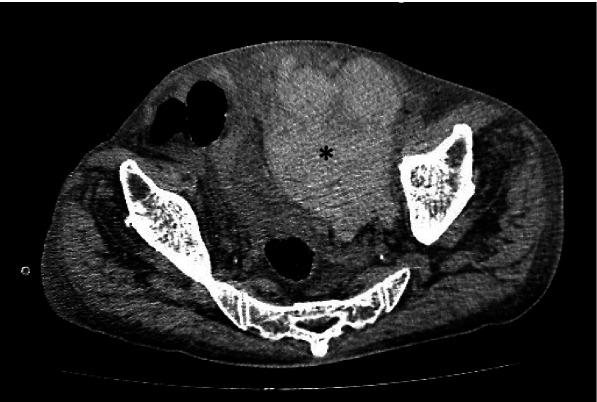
Plain CT abdomen: A hyperdense non-enhancing mass lesion (*) is noted involving the left anterior abdominal wall at the rectus sheath, extending into the prevesical space, measuring at least 10.2 cm × 8.5 cm × 17.3 cm (AP x W x CC) representing a hematoma
